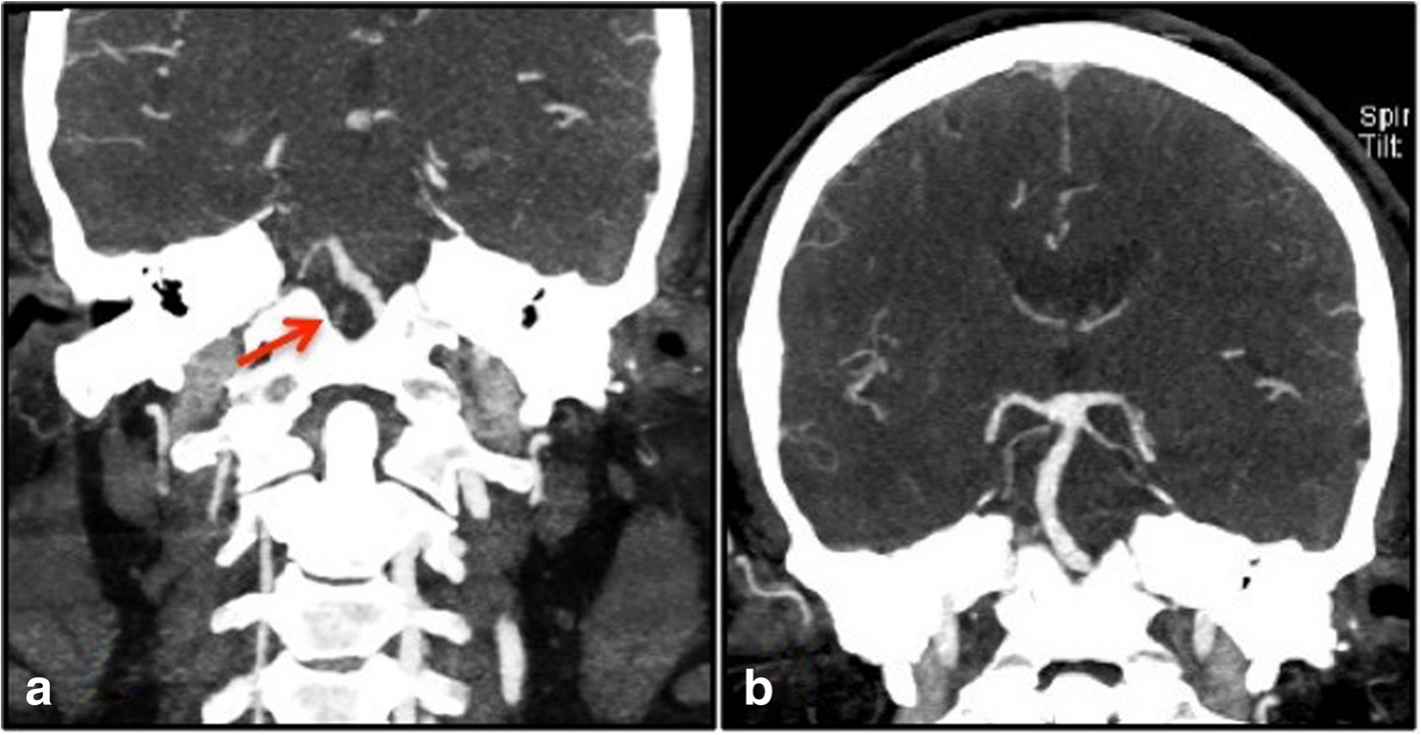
Computed tomographic angiogram of the head and neck coronal sections. a Severe atherosclerotic stenosis of the right vertebral artery at the V4 segment with complete occlusion prior to the formation of the basilar artery (*red arrow*). b Basilar tip sessile aneurysm giving off bilateral posterior cerebral and superior cerebellar arteries
